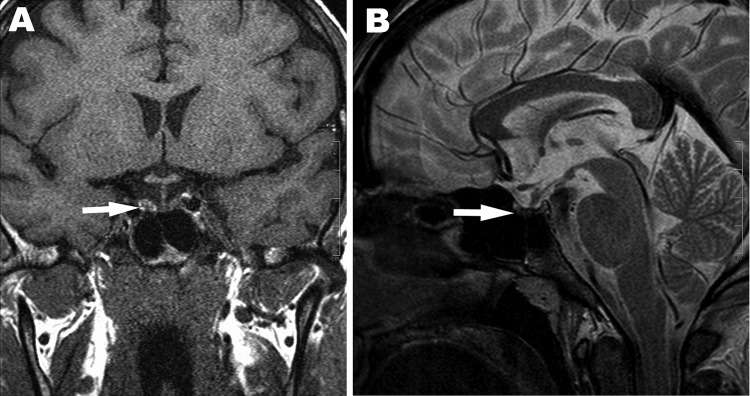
Magnetic resonance images showing hemorrhage of the pituitary gland and pituitary atrophy as indicated by arrows. A) T1-weighted coronal image shows high signal intensity on the right side of the adenohypophysis consistent with hemorrhage. B) T2-weighted sagittal image shows decreased pituitary gland height and heterogenous low signal intensity of the central adenohypophysis due to hemorrhagic infarction.
